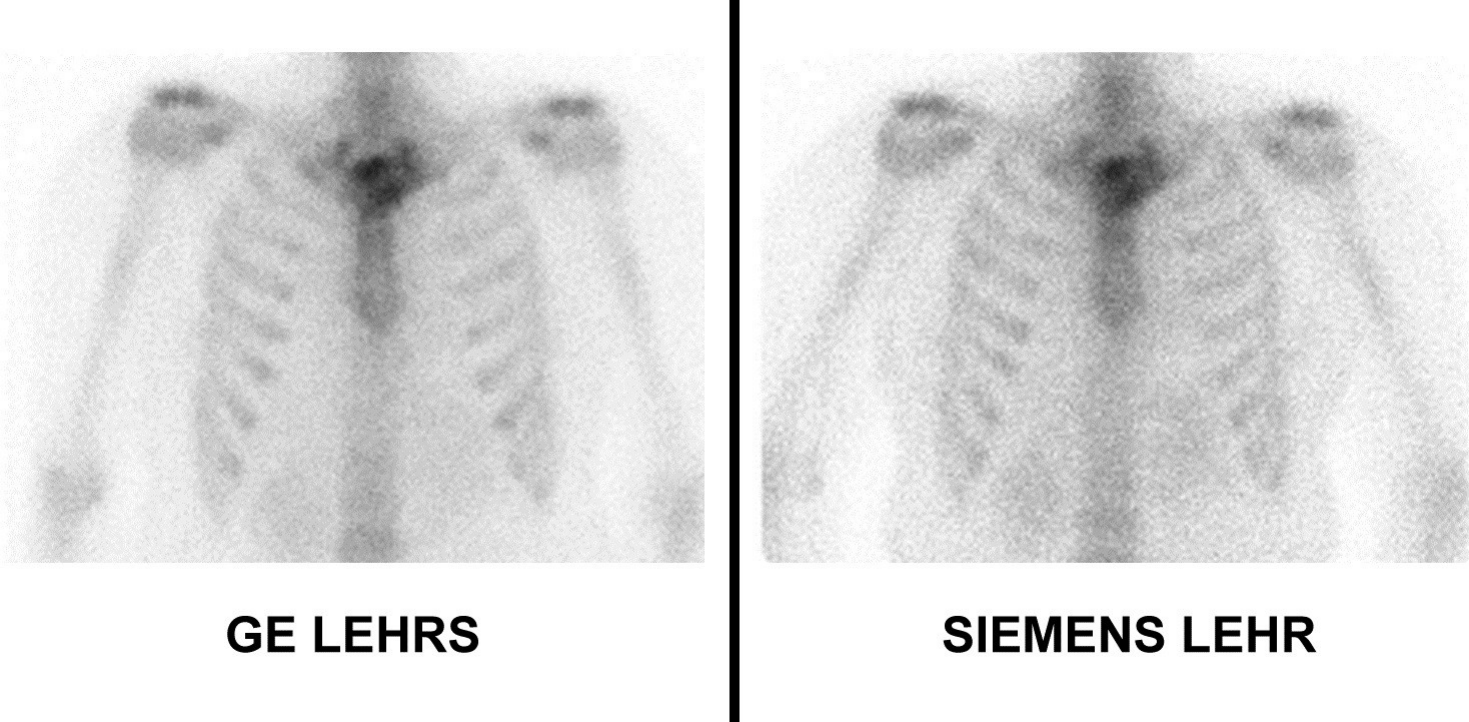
Planar acquisitions in bone scintigraphy.
